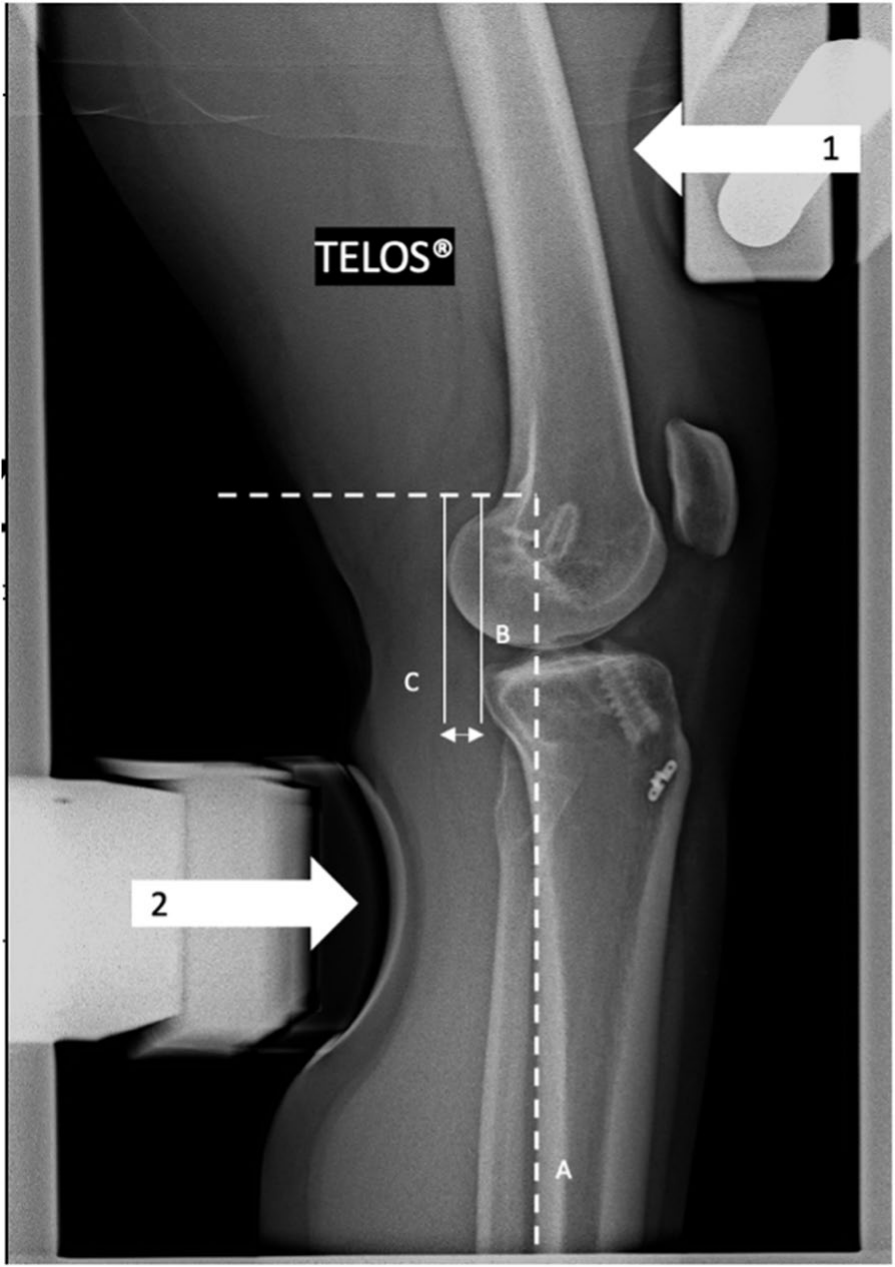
Lateral right knee radiograph demonstrating Dynamic Anterior Tibial Translation (DATT). Measurement of DATT in Telos™ procedure x-rays, using 150N force applying on the femur (arrow 1) and the tibia (arrow 2), on opposite direction. The posterior tibial cortex is the reference (line A). Two lines are traced parallel to line A and tangent to posterior part of the medial plateau (line B) and medial femoral condyle (line C). SATT is the distance between line B and C
